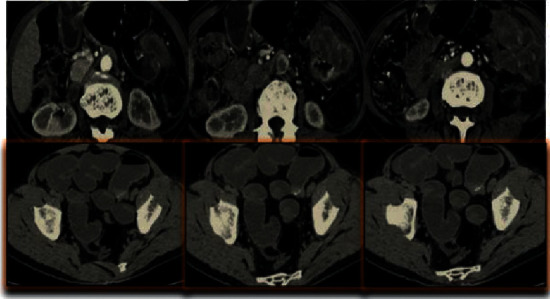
CT images of the patients before being processed by the single-axis super-resolution algorithm. All were enhanced arterial-phase scanning images.
